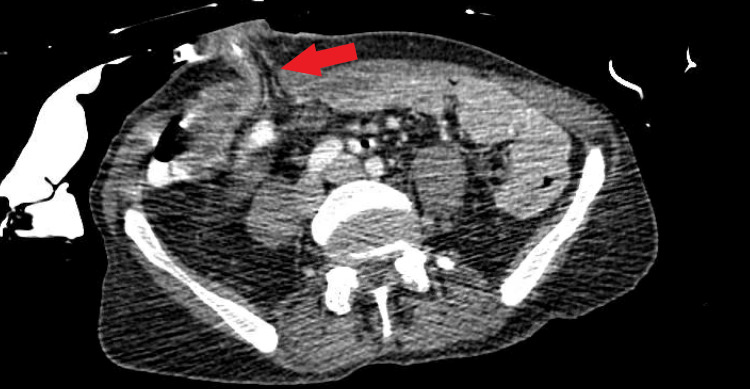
Ileostomy shown in CT of the abdomen and pelvis
